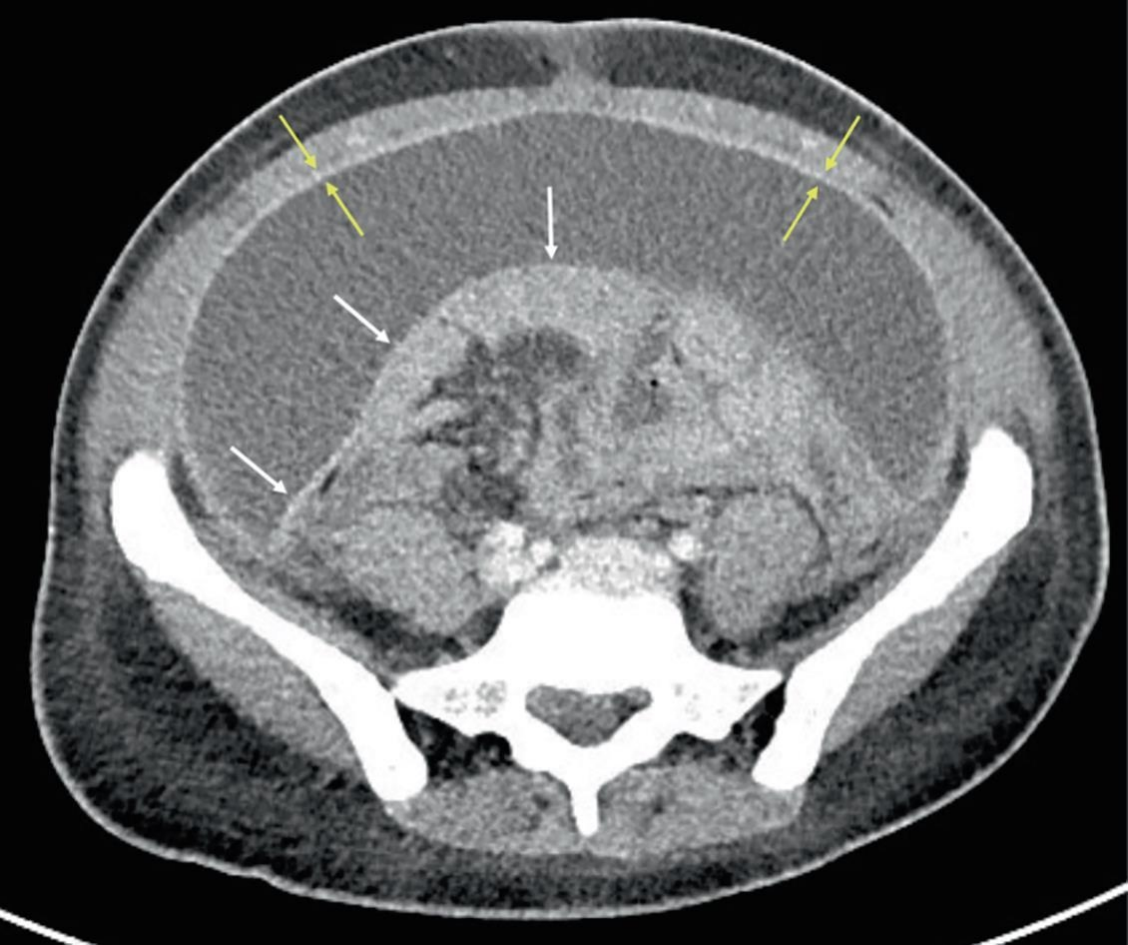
Contrast‐enhanced CT revealing thickening of parietal peritoneum (yellow arrows), and development of coalescent sheet‐like fibrotic tissue (white arrows) surrounding the entire bowel and mesentery, forming a “cocoon” in the central abdomen.
